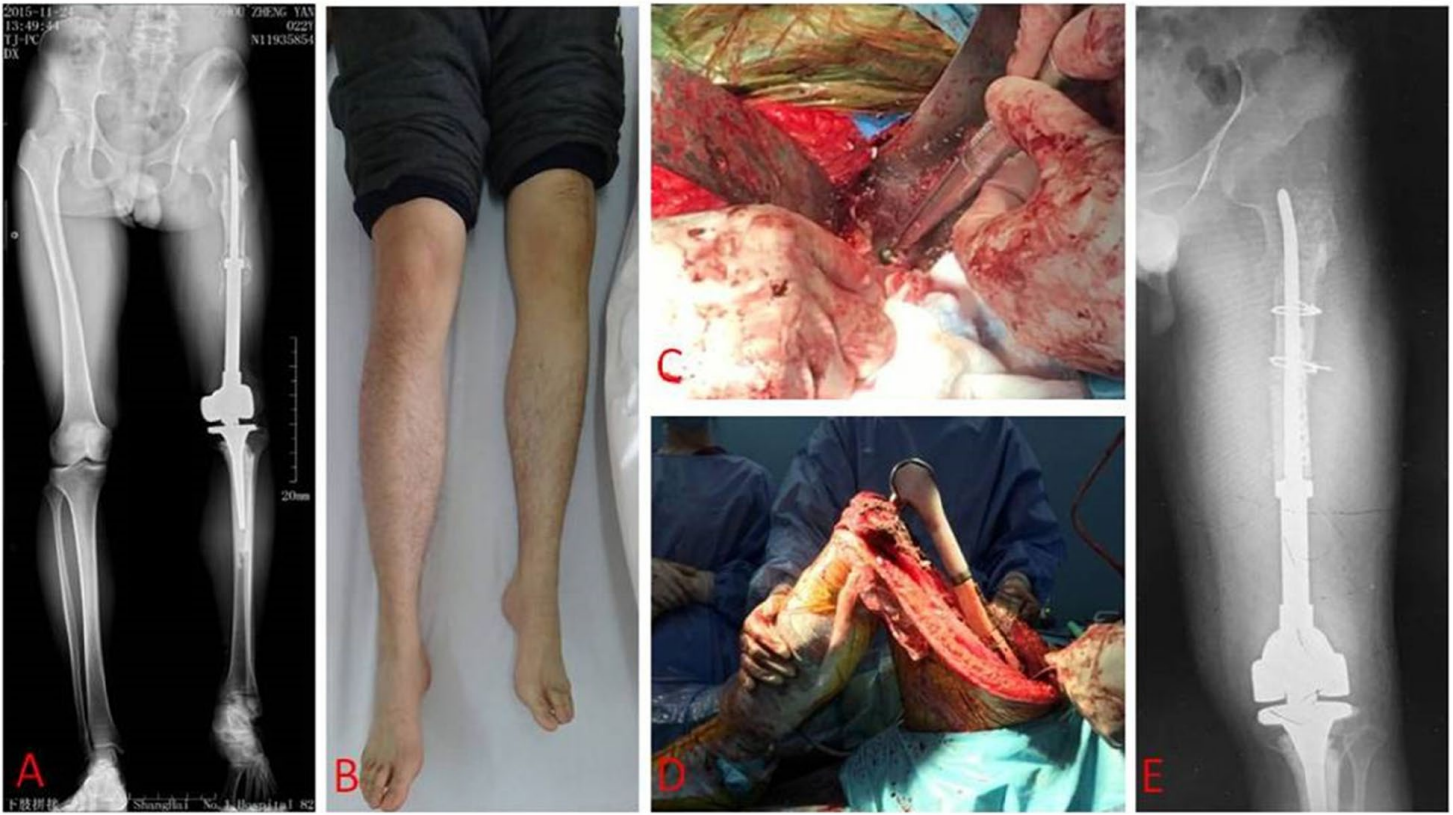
A 57-year-old male osteosarcoma patient, 14 years after initial endoprosthetic replacement. A Preoperative X-ray showed proximal femur prosthetic component loosening and breakout. B The shortened affected extremity, leg length discrepancy (LLD). C Allograft segment, two cables, plate, and screws were used to reconstruct and fix the proximal prostheses. D Proximal component was rebuilt. E, postoperative 1-year X-ray showed prostheses and other components in position
